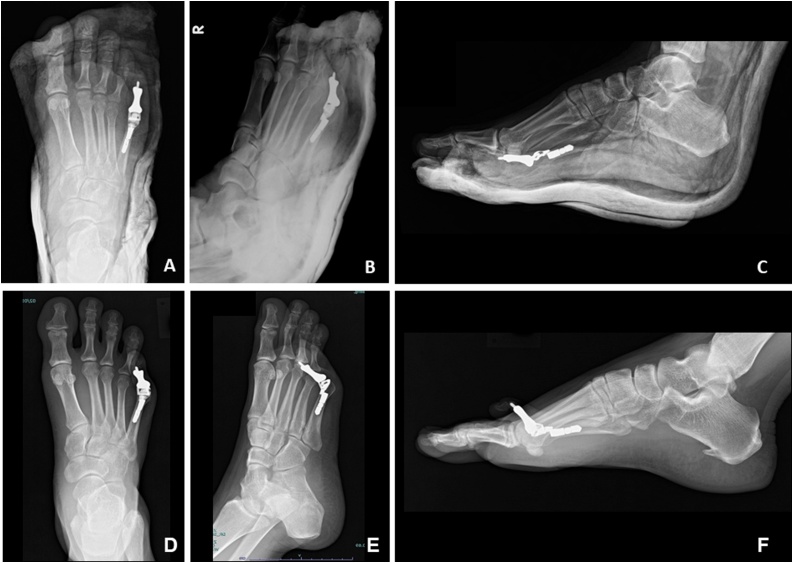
Immediate postoperative images. Anteroposterior (4A), oblique (4B), and lateral (4C) radiographs of the right foot show proper prosthesis placement. Subsequently, overriding toe occurred in two years and evidenced in AP (4D), oblique (4E) and lateral (4 F) radiographs due to scar contracture.
